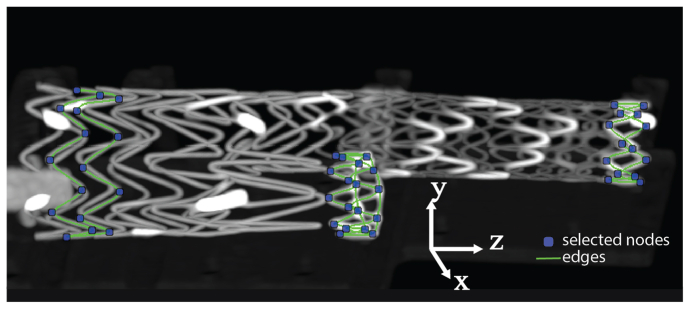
Maximal intensity projection of a phase-averaged computed tomography (CT) volume of the stent graft in the setup. The models resulting from the segmentation of the three individual stent rings are visualized as nodes (blue) and edges (green). The nodes were used to calculate the motion amplitudes and path lengths. The edges connect the nodes over the stent wire frame.
